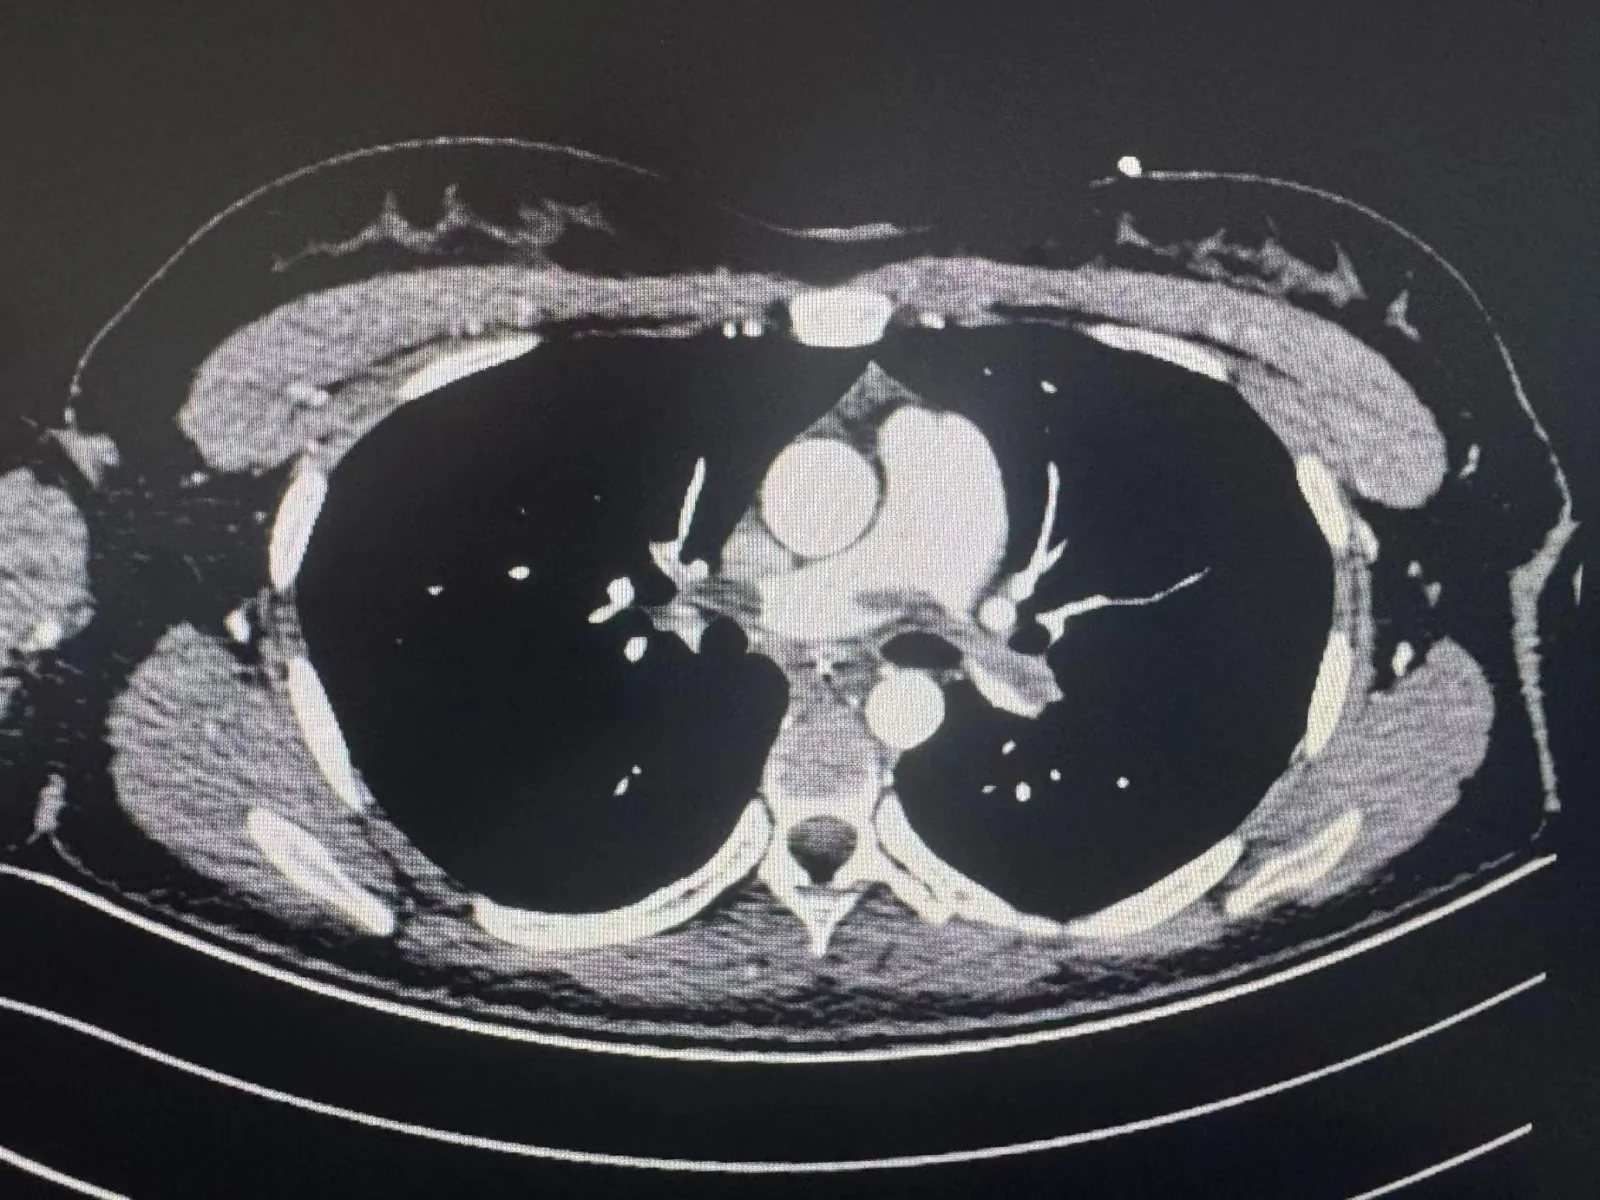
CTPA after admission demonstrating a saddle embolus at the bifurcation of the main pulmonary artery. CTPA = computed tomography pulmonary angiography.
